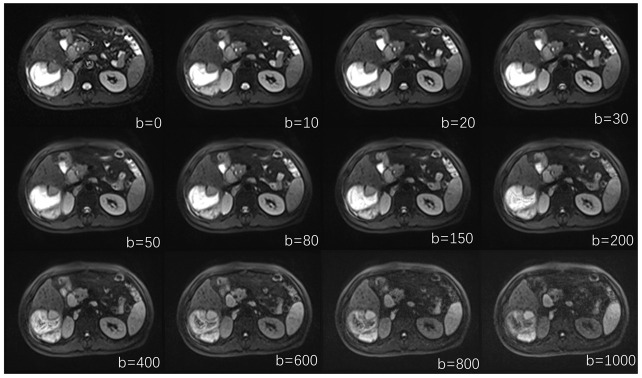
IVIM images at different b values. IVIM-DWI images with 12 b-values, representing b values of 0, 10, 20, 30, 50, 80, 150, 200, 400, 600, 800, and 1000 s/mm^2^.
